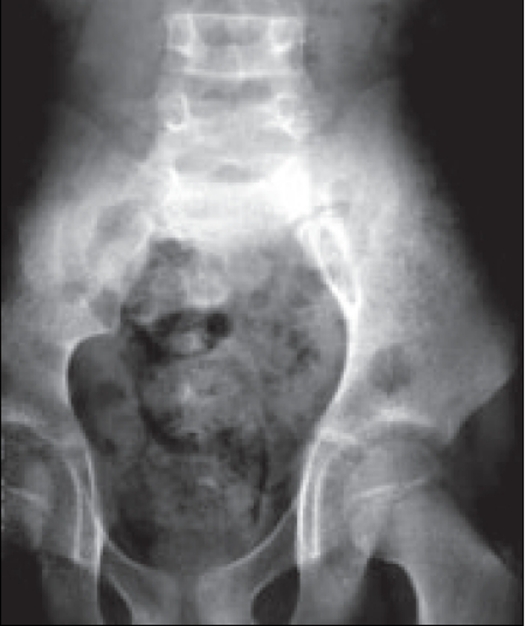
Frontal radiograph showing absence of left sacrum(Scimitar sacrum)-Case 2
